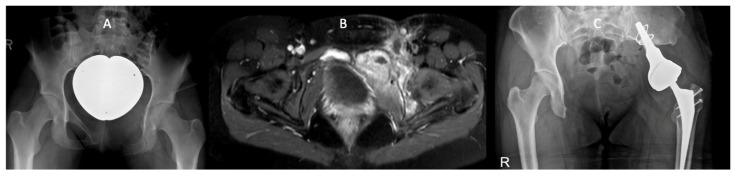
Example Case; Image (A) shows the preoperative finding of the Ewing sarcoma in a pelvis overview radiograph. Image (B) depicts the tumor spread in an axial MRI scan. Image (C) shows the latest pelvis overview radiograph after the implantation of the tumor endoprosthesis.
